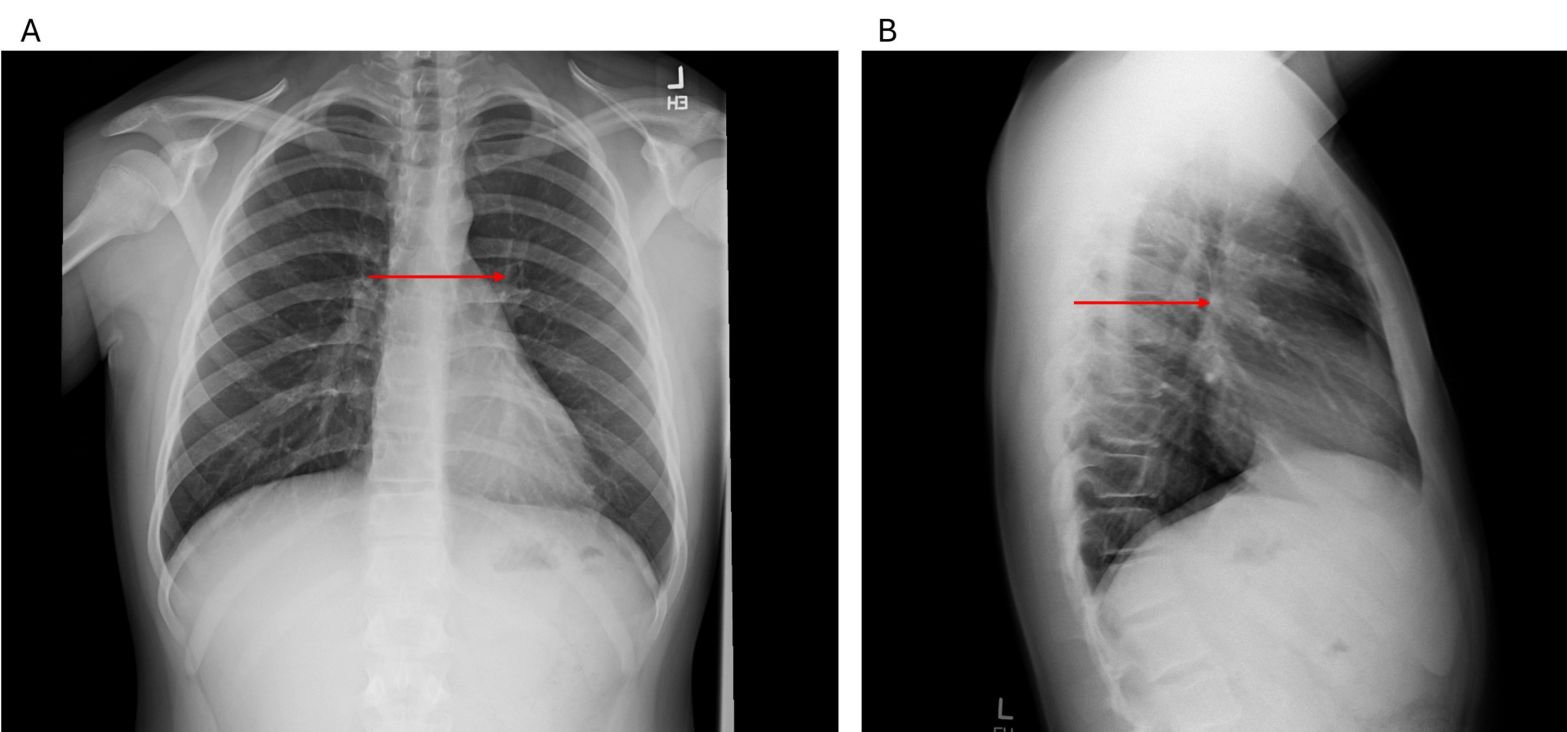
Follow-up posteroanterior (A) and lateral (B) chest X-ray after recovery. Arrows indicate previously affected areas now resolved.
